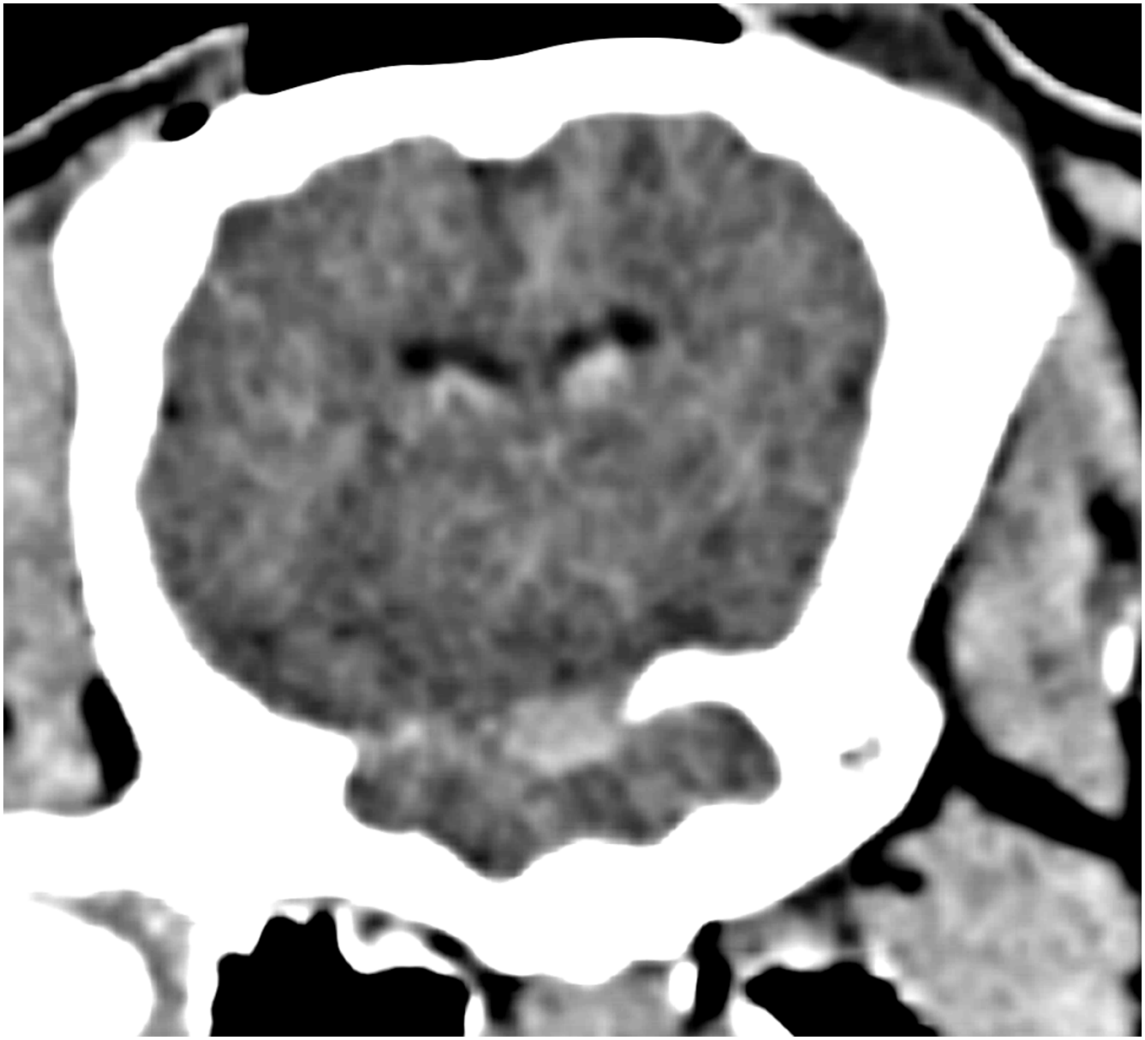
CT image after experiment verifying blood distribution in the basal cisterns. Blood is also visible in the lateral ventricles. Image width 7
